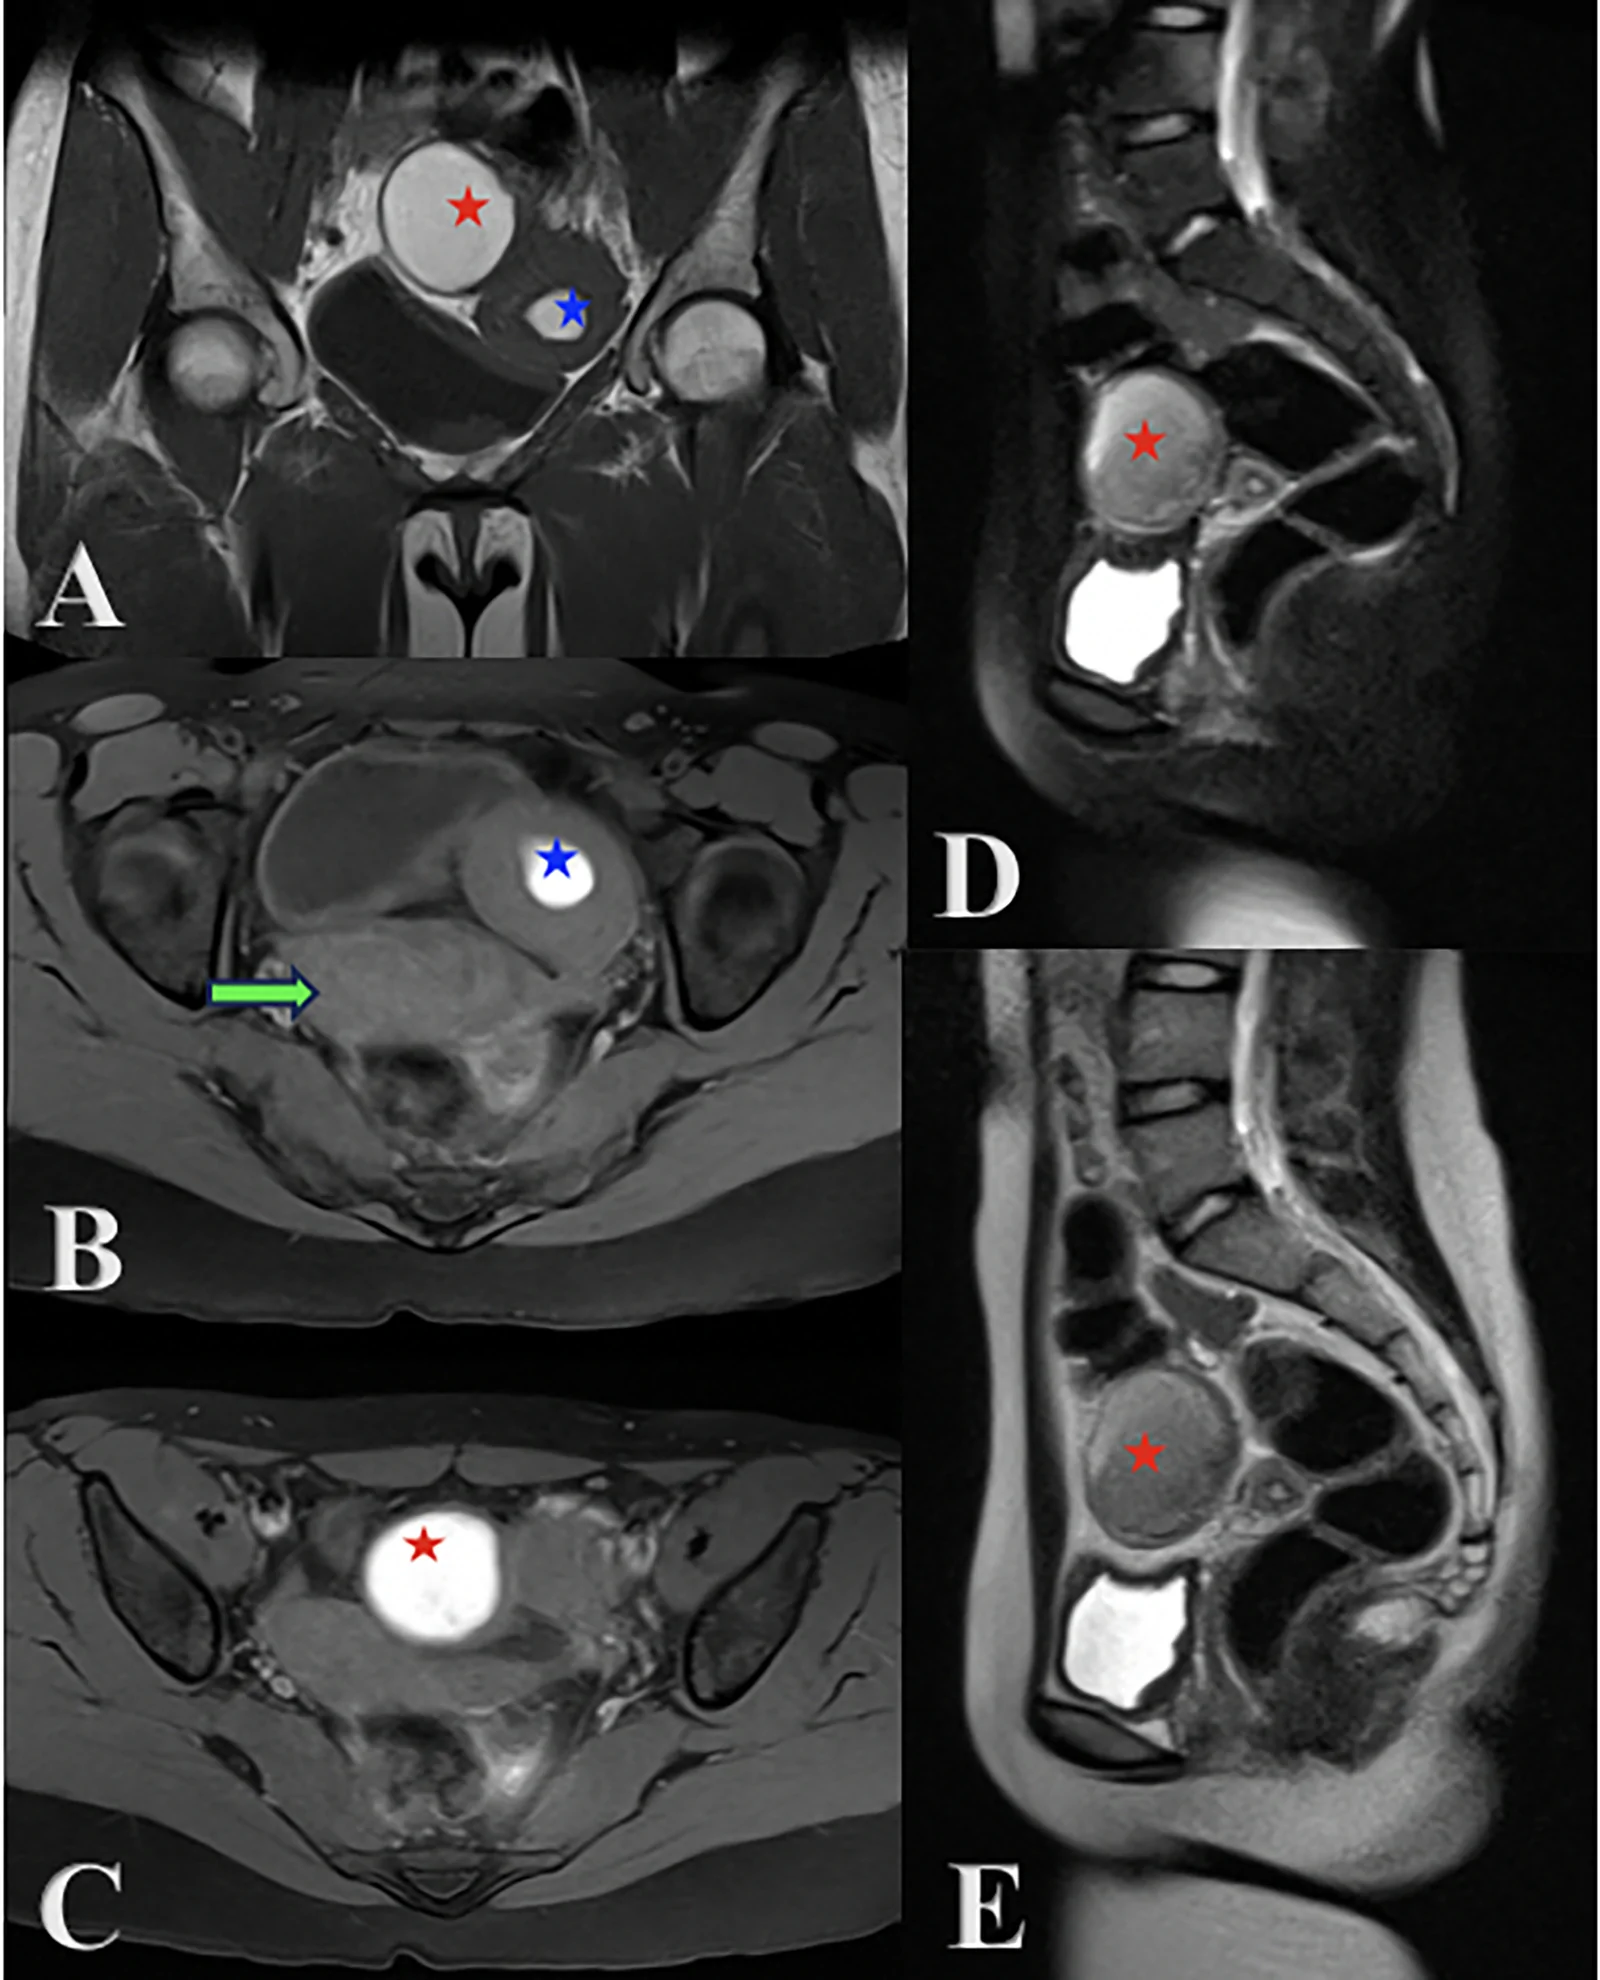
MRI features of hematometra and left ovarian endometrioma. (A) Coronal T1-weighted image, (B–C) Axial T1-weighted fat-suppressed images, and (D–E) Sagittal T2-weighted fat-suppressed and T2-weighted images. The left distended endometrial cavity (marked with a blue star) demonstrates high signal intensity on T1-weighted and T1 fat-suppressed images, consistent with hematometra. A cystic lesion in the left adnexal region (marked with a red star) demonstrates high signal intensity on T1-weighted images with corresponding low signal intensity on T2-weighted sequences, representing the characteristic shading sign, which reflects chronic blood products containing deoxyhemoglobin and methemoglobin, a hallmark feature of an ovarian endometrioma. The right uterine unit (marked with a green arrow) appears normal without evidence of obstruction.
